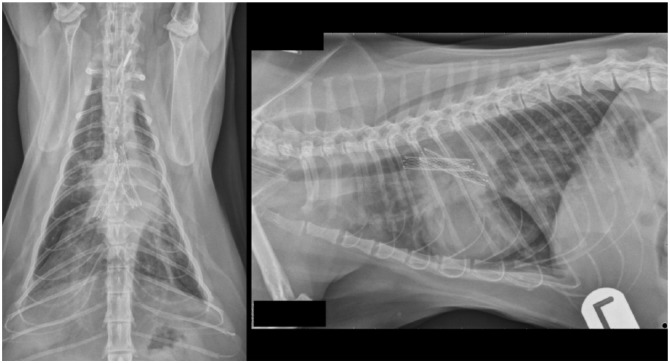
Dorsoventral and left lateral radiographic projections obtained 4 weeks after stent deployment, obtained to investigate worsening of respiratory signs. An interstitial-alveolar pattern is visible diffusely in the lung fields, worst in the right caudal lobe. Stent position and conformation was appropriate, with no evidence of migration or fracture
